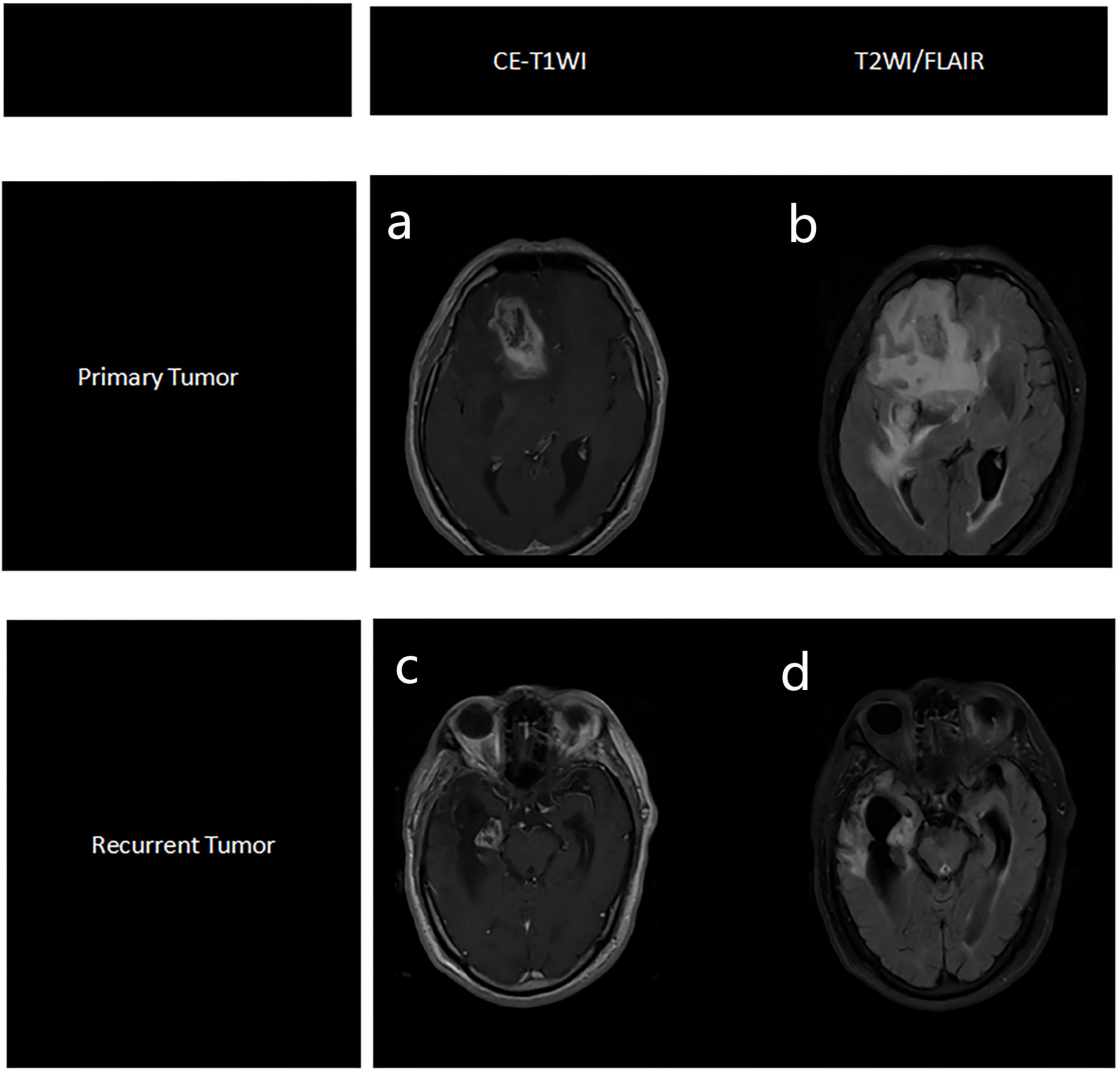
Representative MRI images of primary tumor, based on CE-T1WI sequence (a) and T2WI/FLAIR sequence (b), and recurrent tumors, based on CE-T1WI sequence (c) and T2WI/FLAIR sequence (d).
